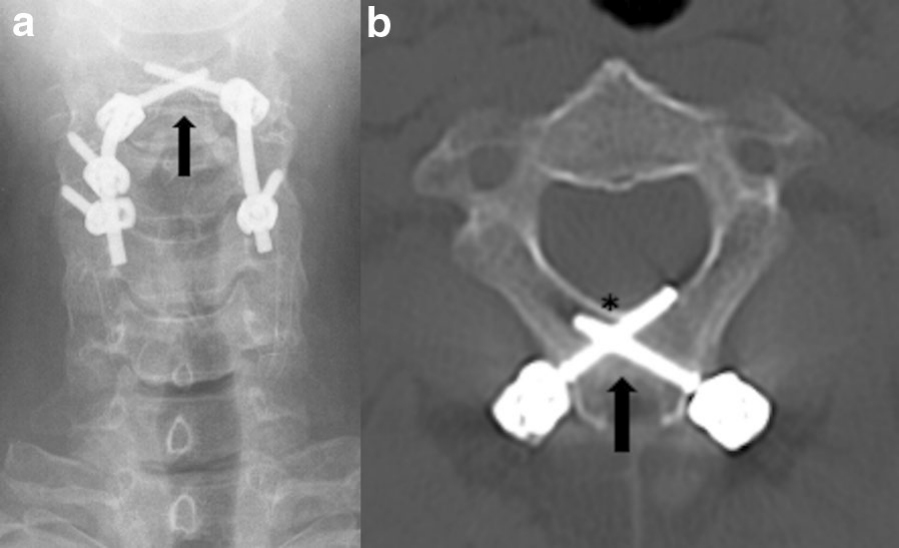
Rods with translaminar screws—posterior stabilization procedure due to trauma (fracture and a mild traumatic anterolisthesis). Anteroposterior radiograph (a) and axial CT image (b) demonstrate bilateral crossing C2 laminar screws (black arrows). Although the right screw is tangent to the inner cortex (asterisk), this positioning is acceptable
